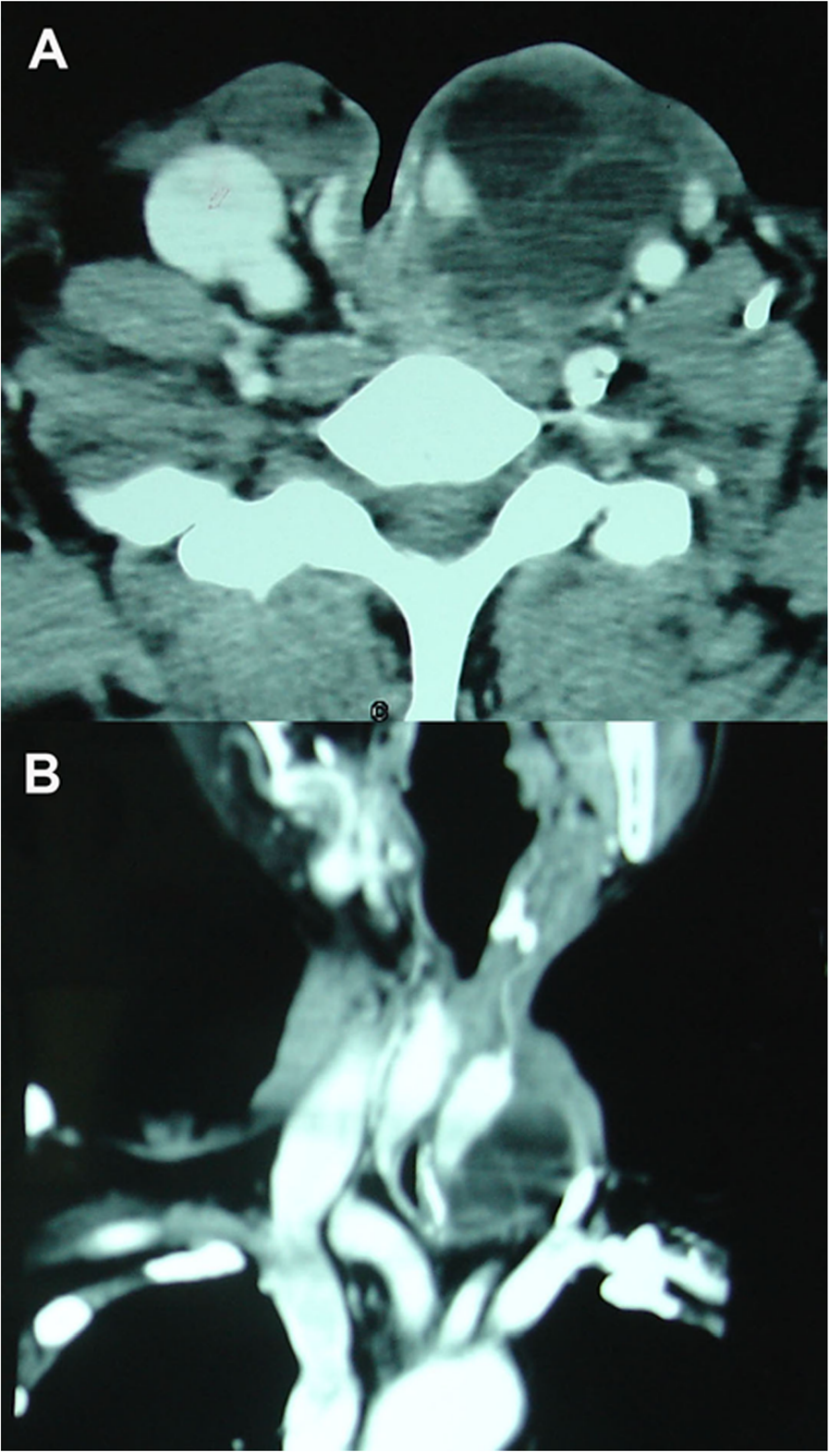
Axial (a) and coronal (b) computed tomography scans showed a well-circumscribed, non-enhancing, cystic-solid mass in the left side of the tracheal stoma
